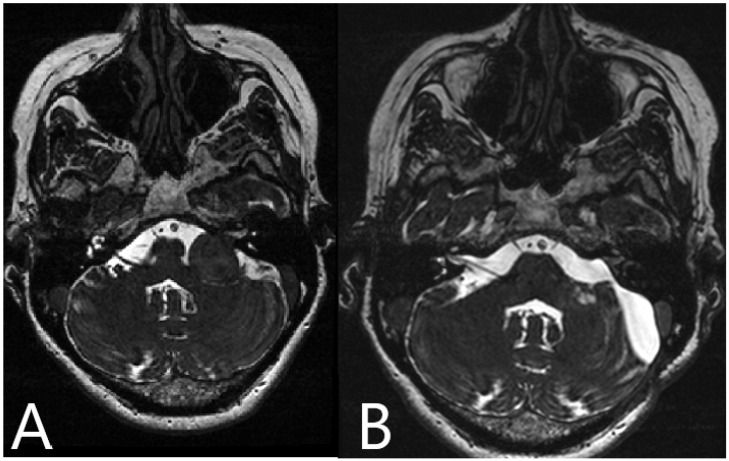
Patient No. 34. (A) Preoperative and (B) postoperative T2-weighted MRI of the head shows subtotal resection of the left sided AN.
